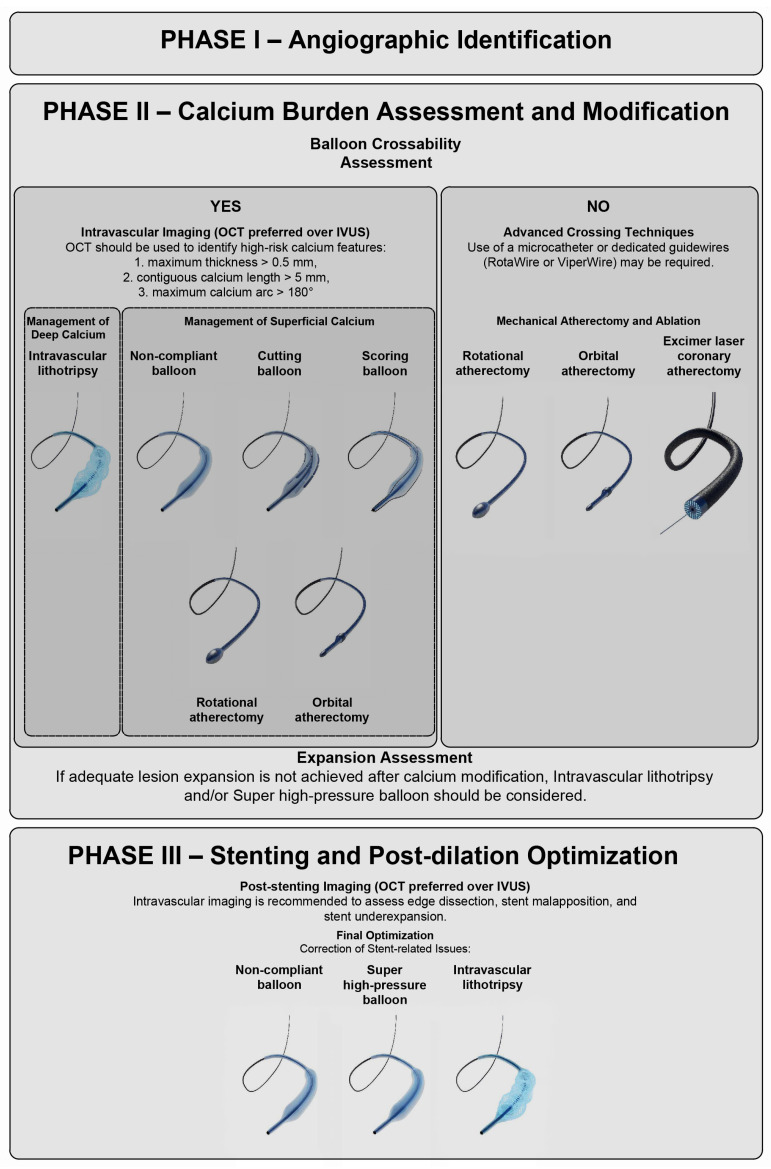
Algorithm for the management of calcified coronary lesions. OCT—optical coherence tomography; IVUS—intravascular ultrasound.
